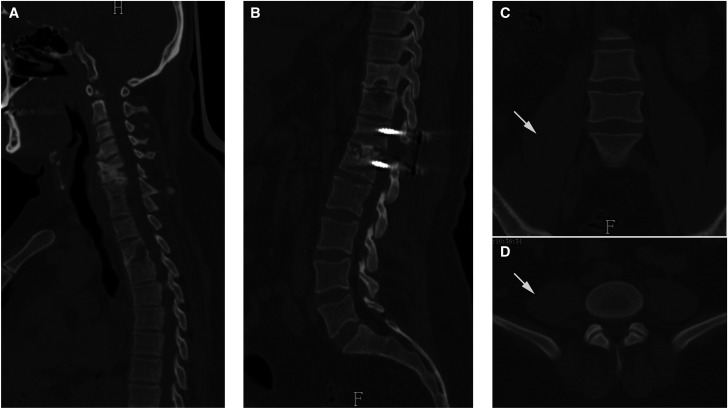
CT images at 12-month follow-up. (A and B) Sagittal CT images suggest the bony destruction improved in the C5, C6, T3, T10, T12, and L1 vertebrae, and bony fusion was achieved between the T12 and L1 vertebrae. (C and D) Coronal and axial CT scans indicate that the psoas abscess was cured (white arrows). CT = computed tomography.
